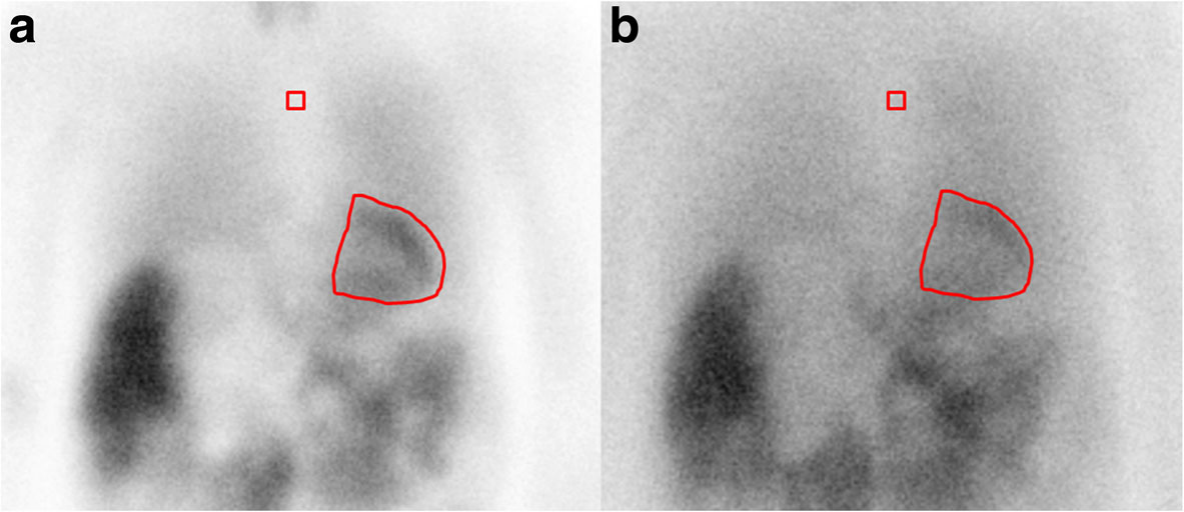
Planar images from ^123^I-MIBG scintigraphy of a patient during chronic HD. a Early image 15 min after tracer administration; b late image 4 h after tracer administration. Late heart-to-mediastinum ratio (HMR) is the ratio between the counts within a reference point in the upper mediastinum (*red square box*) and the left ventricle (*red contoured region*). The HMR of panel a was 3.03 and of panel b 1.80, resulting in a 41% washout
